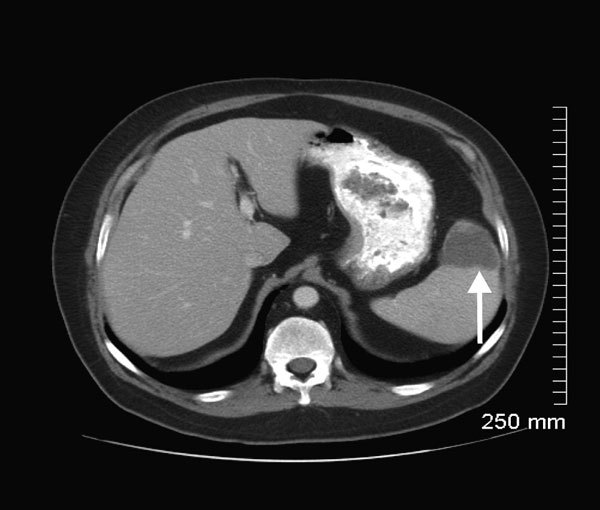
Computed tomography (CT) scan of the abdomen of patient B, a renal transplant recipient infected with *Babesia microti* parasites, Wisconsin, USA, 2008. Taken on November 5, the scan shows a splenic infarction (white arrow) that had not been visualized on a CT scan on October 5. Although the cause of the splenic infarction was not determined, the infarction might have been a complication of babesiosis, as reported for other patients (*16,17*).
